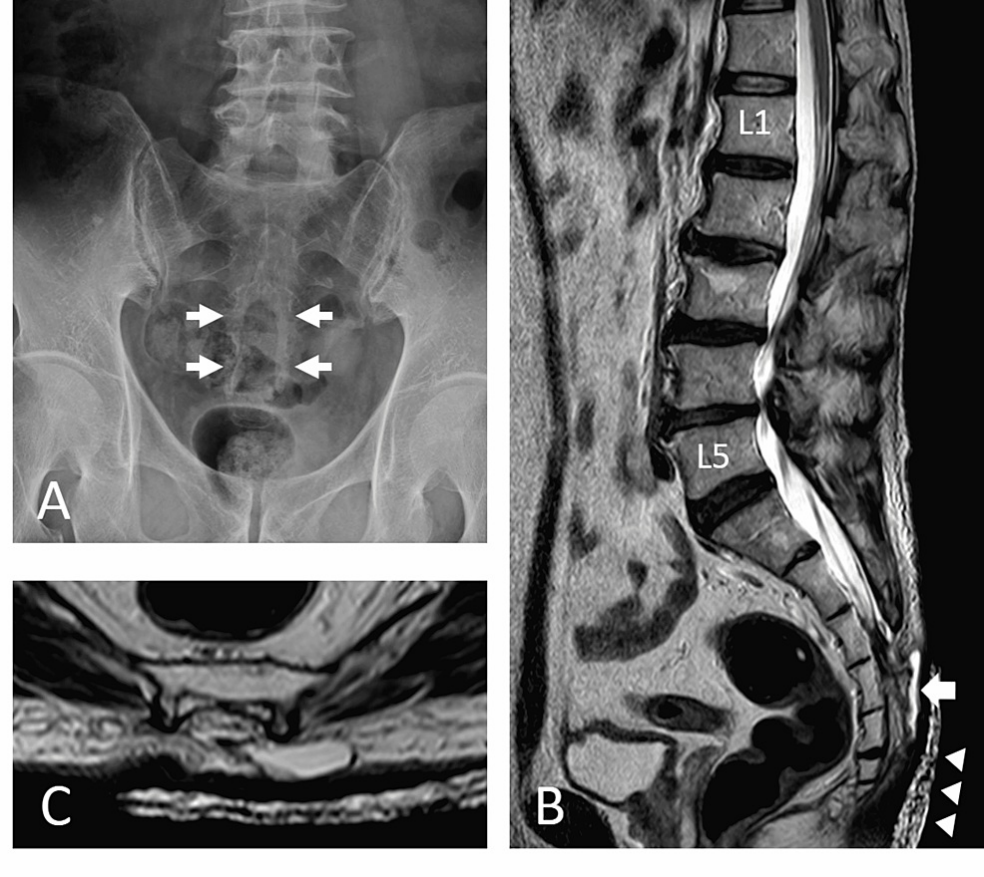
Radiographic image findings. (A) Lumbosacral spine X-ray radiography revealed spina bifida at S3 and below (arrow). (B, C) Magnetic resonance imaging revealed a cyst-like structure (arrow) under the skin at the S3–S5 level. The terminal end of the dural sac and soft tissue contrast were continuous. Tethered cord and lipoma were not detected. Arrowhead: water-absorbing sheet.
